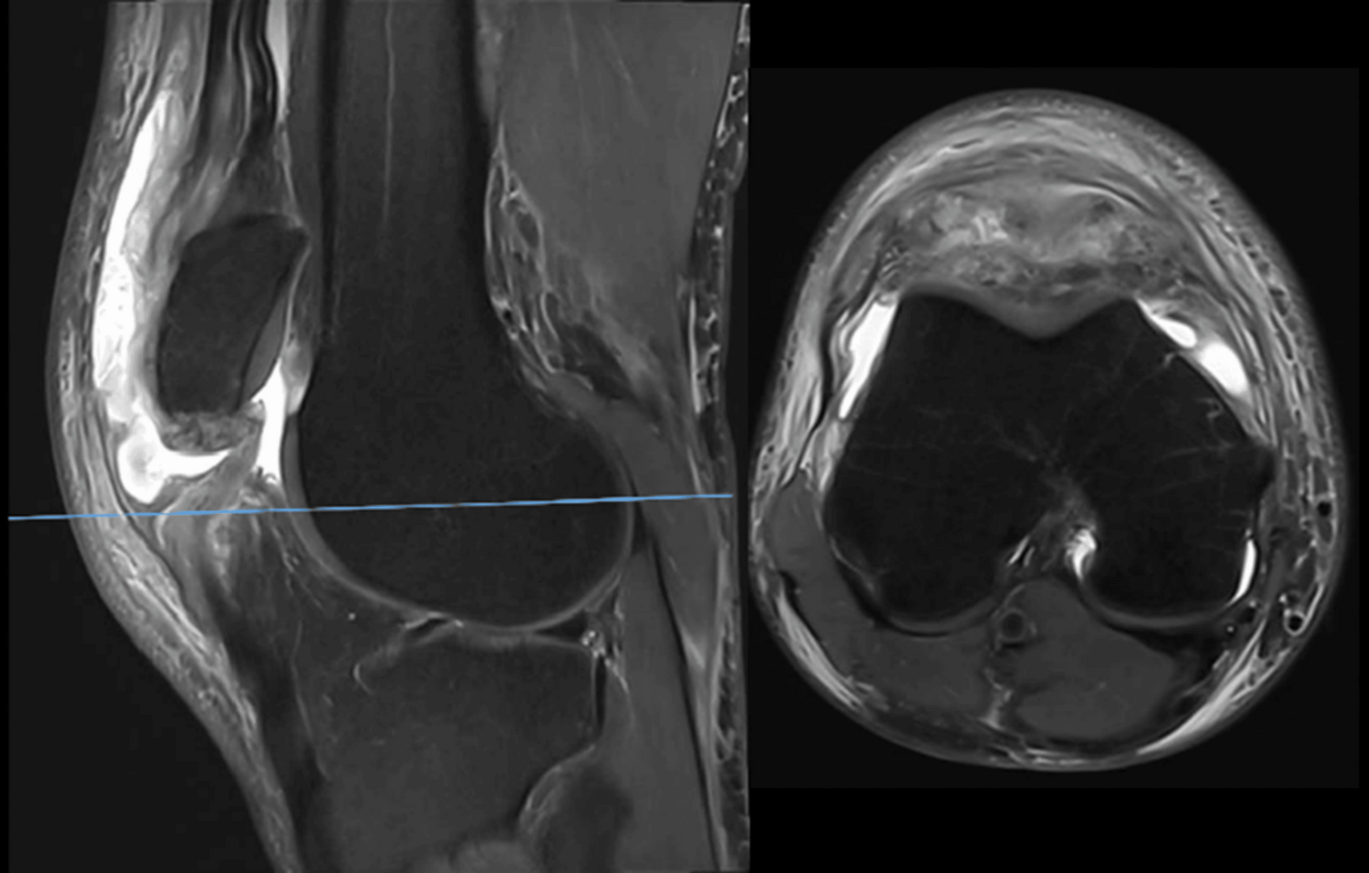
Sagittal and axial T2-weighted MRI images at second presentation, demonstrating deficient extensor mechanism function. The proximal patellar tendon fibers are discontinuous, consistent with rupture. MRI, magnetic resonance imaging
